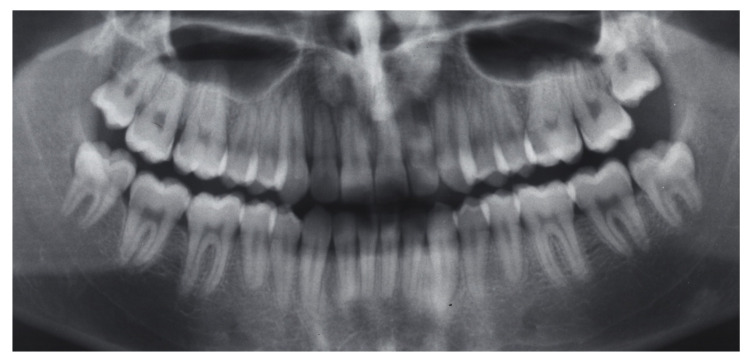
Post-treatment orthopantomogram.
